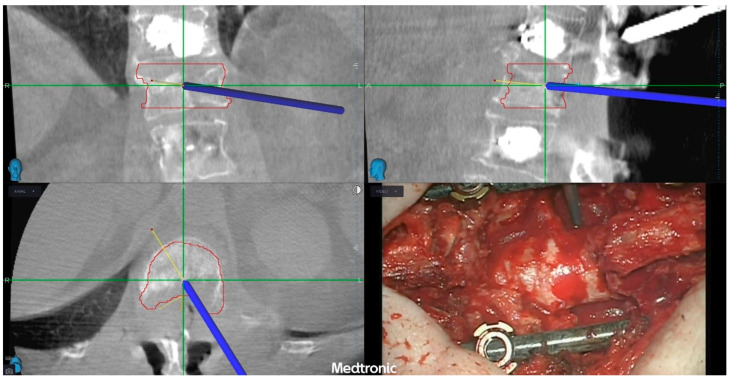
Final check after rod placement: given the potential for tumor regrowth and the evident manual merging error—partly due to the absence of an adequate volumetric lumbar CT/MRI imaging—the resection margin extends deliberately slightly beyond the minimum SBRT-target boundary.
